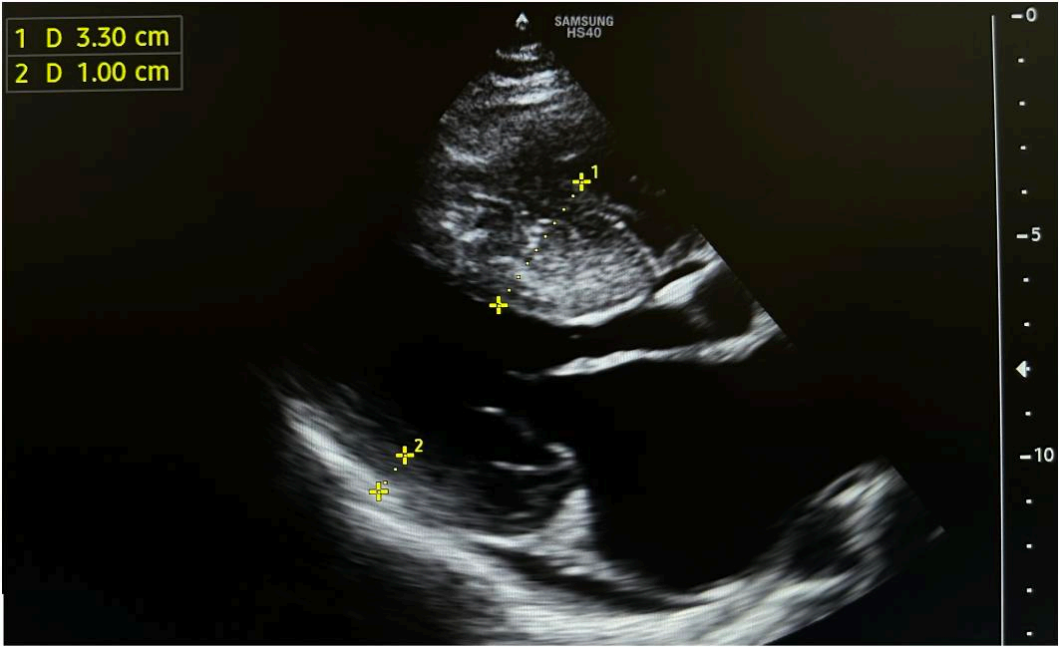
The initial echocardiography conducted prior to the ethanol ablation procedure revealed a thickened left ventricular septum, measuring up to 33 mm, causing obstruction within the left ventricular outflow tract up to 40 mmHg of gradient.
